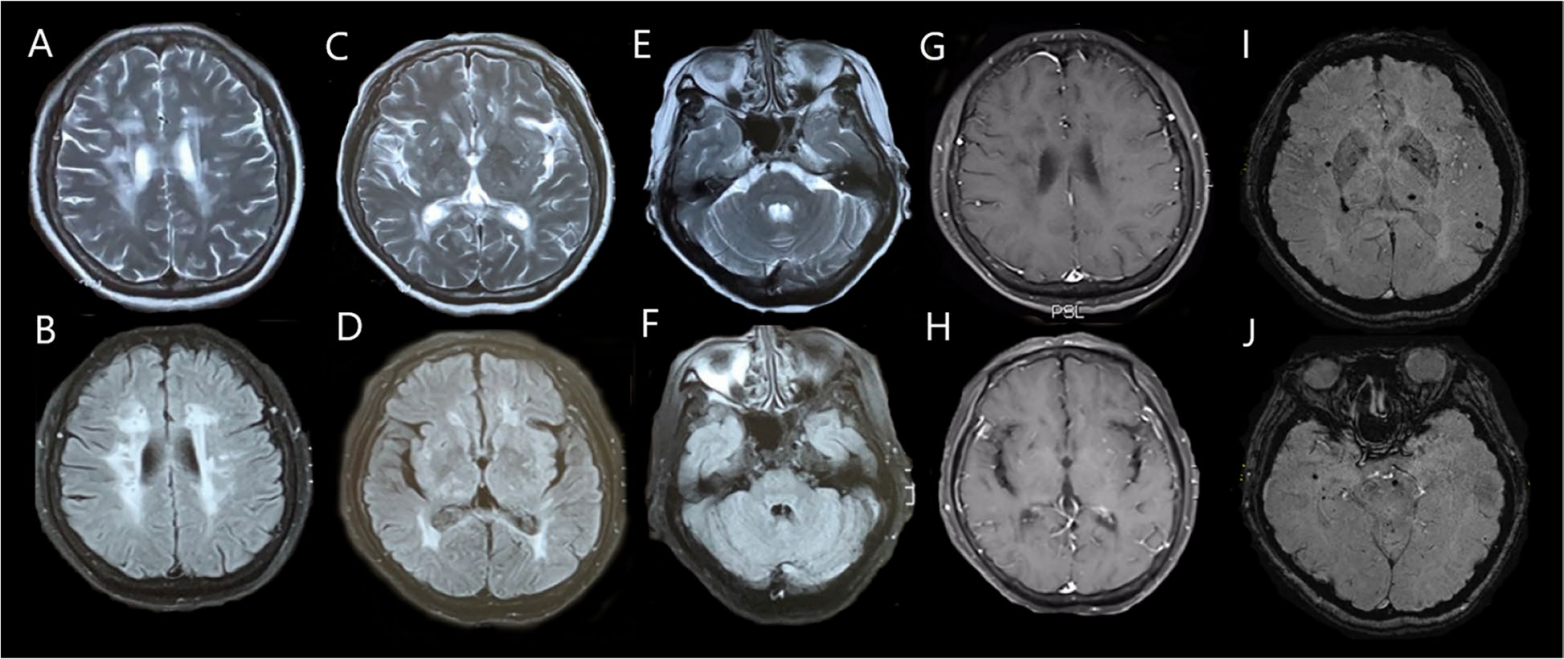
Brain MRI of the patient in May 2018T2-weighted (A, C, E) and FLAIR images (B, D, F) showed multiple lacunar infarcts throughout the bilateral basal ganglia, thalamus, periventricular white matter and subcortical white matter. Enhanced scan showed no obvious enhancement (G, H). Susceptibility-weighted imaging showed the multiple cerebral microbleeds in the basal ganglia, brain stem and subcortical areas (I, J)
